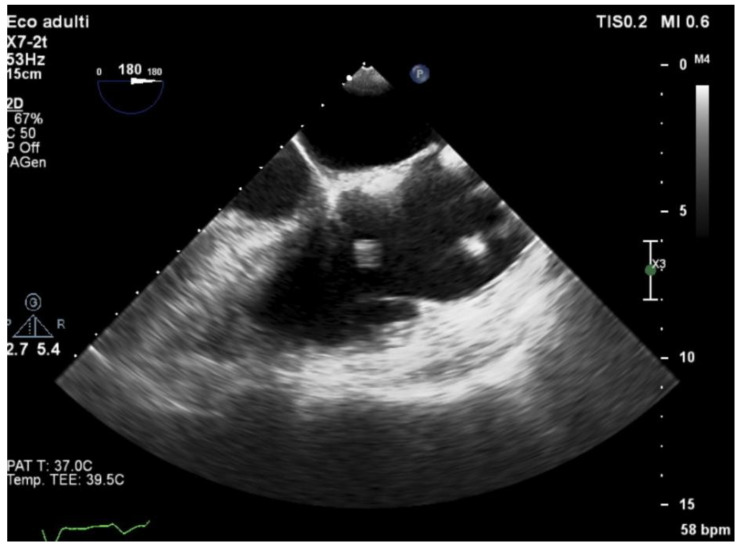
Transesophageal echocardiography—midesophageal RV-focused view: vegetating lesions attached to the right atrial portion of the pacemaker lead.
